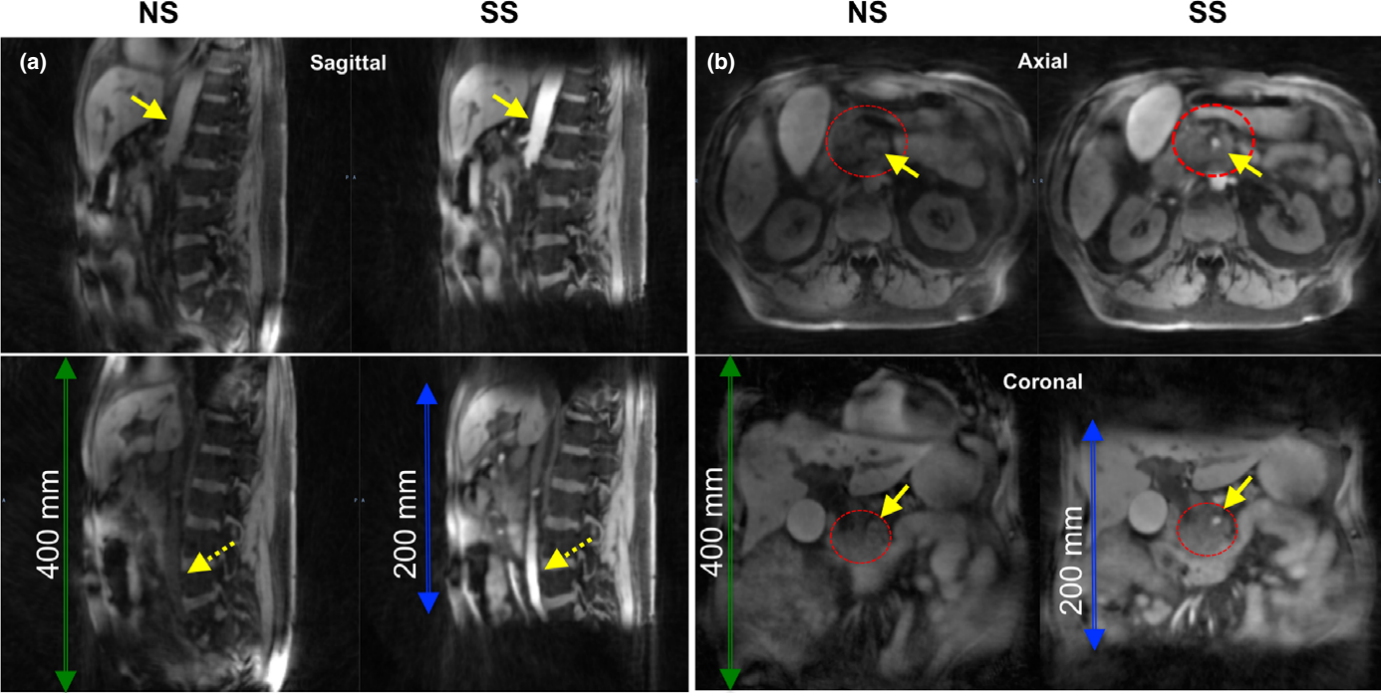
(a) Large blood vessel (aorta (solid arrow) and inferior vena cava (dashed arrow)) to tissue contrast of non‐selective (NS)‐4D‐MRI vs. slab‐selective (SS)‐4D‐MRI in a sagittal view. (b) small blood vessel (superior mesenteric artery, SMA, solid arrows) to tissue contrast of NS‐4D‐MRI vs. SS‐4D‐MRI in an axial and coronal view. The dashed circle denotes the pancreatic tumor location for this particular patient.
